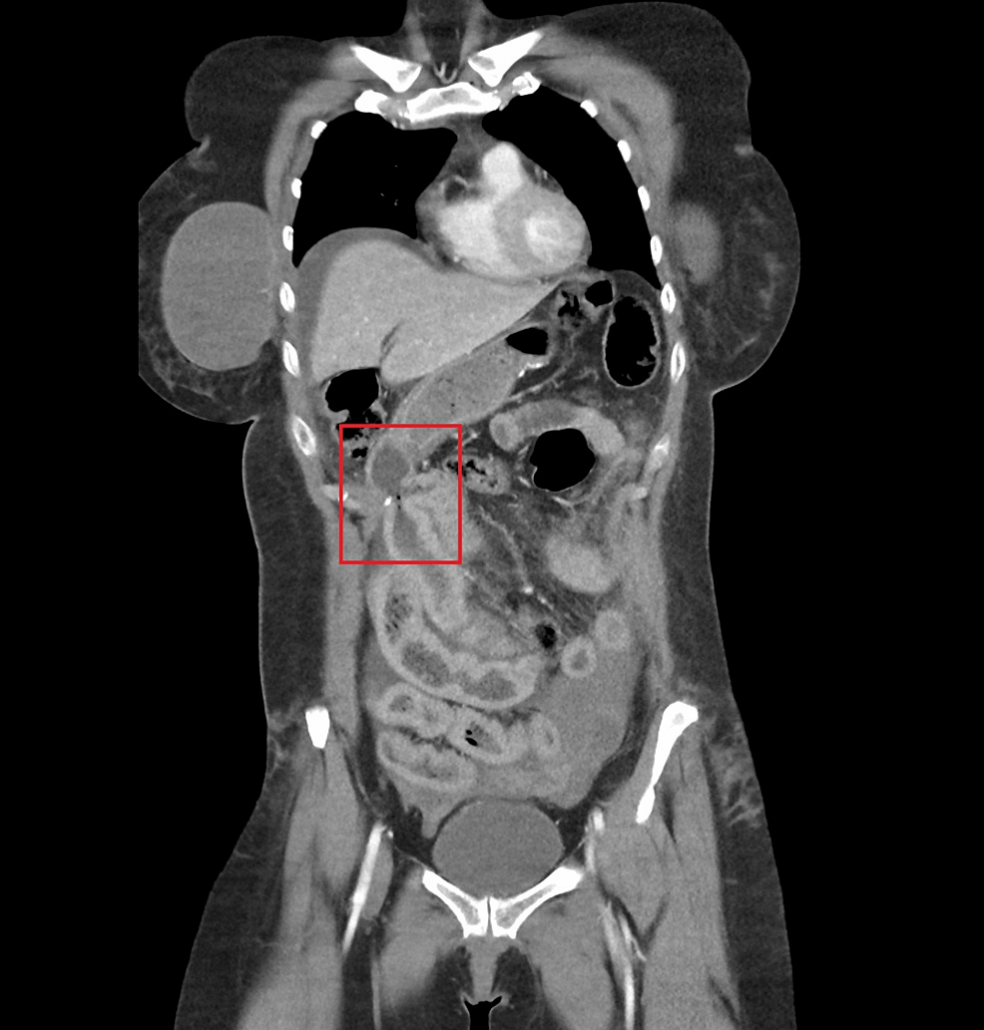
CT abdomen and pelvis scan showing SADI-S gastro-ileostomy CT: computed tomography, SADI-S: single anastomosis duodenal-ileal bypass with sleeve
